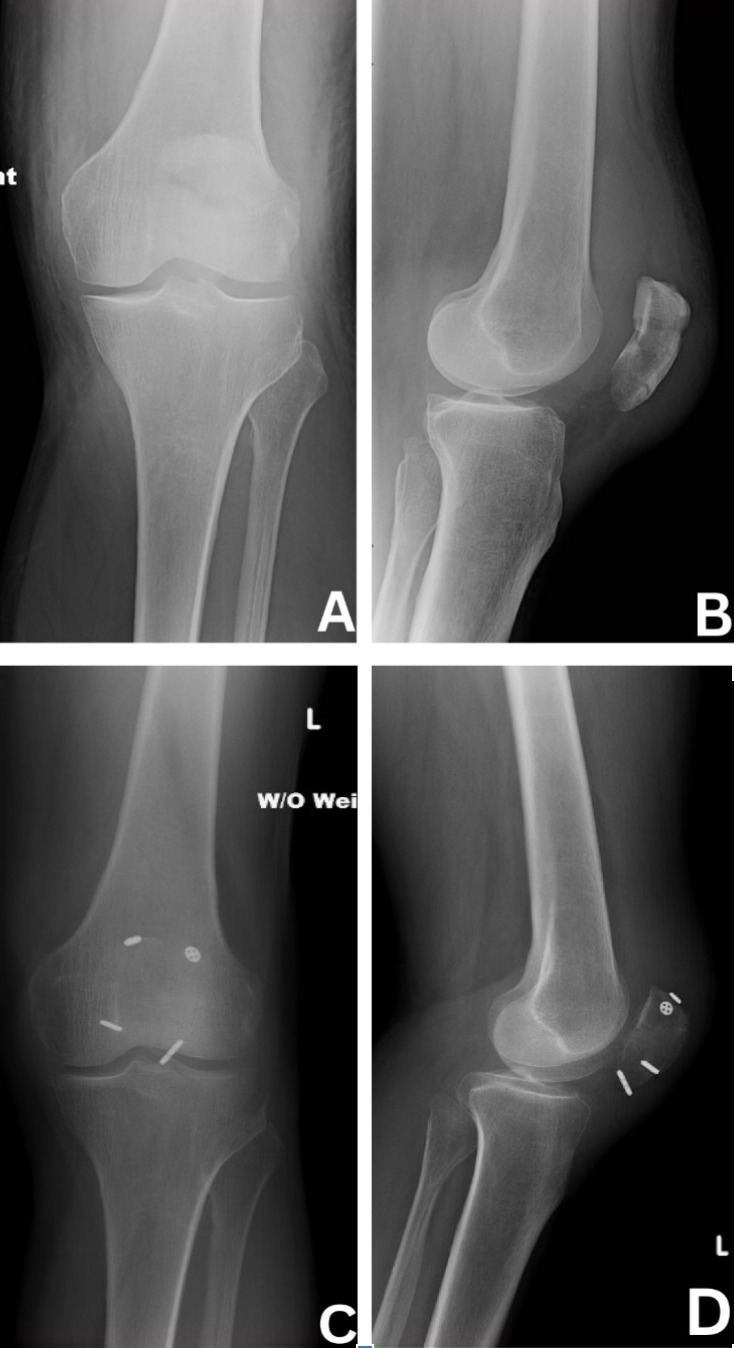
A and B demonstrate preoperative radiographs of a transverse patella fracture. C and D are postoperative radiographs of successful fixation of the fracture after ORIF using our suture button and suture tension band construct.
